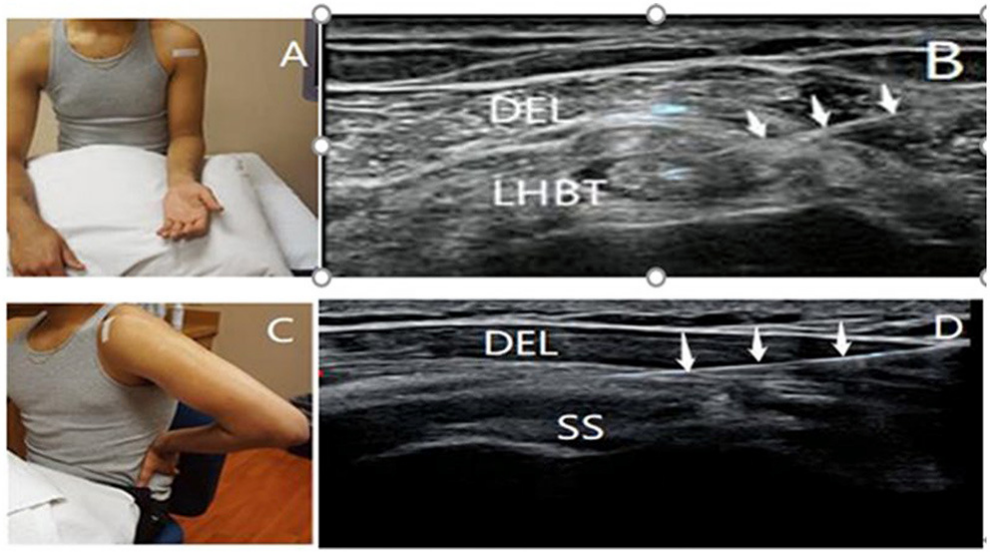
Patient's position and ultrasonography image of SASD bursa and LHBT sheath injection. The patient was positioned to obtain views of the long head of the biceps tendon (A) and subacromial-subdeltoid bursa (C) during the ultrasound-guided injection. The white tape indicates the position of the transducer. Ultrasound images showing the needles inserted into the proximal bicipital tendon sheath (B) and subacromial-subdeltoid bursa (D) (↓, needle; DEL, deltoid; SASD, subacromial-subdeltoid; SS, supraspinatus tendon; LHBT, the long head of the biceps tendon).
